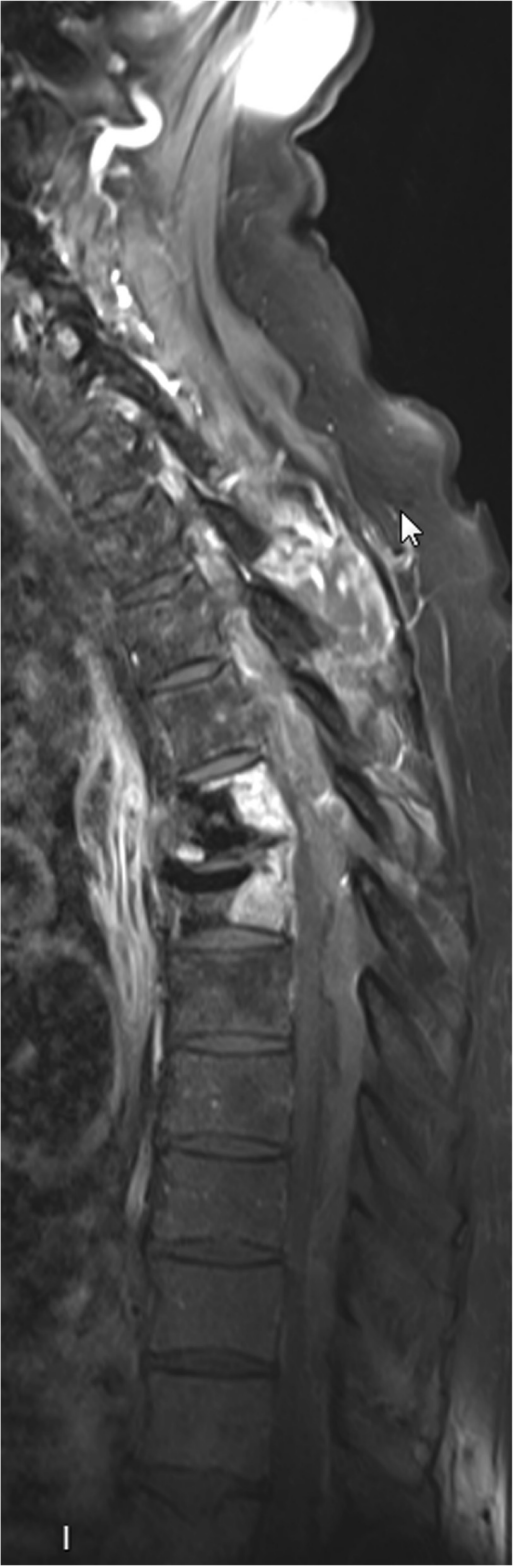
Spine MRI performed after Gadolinium intravenous contrast shows epidural posterior abscess from the third cervical (C3) to the first lumbar vertebra (L1). Medullary compression is seen without medullary suffering. Not well shown in this figure is abscess around the cement (*fifth* and *sixth*) thoracic vertebra
